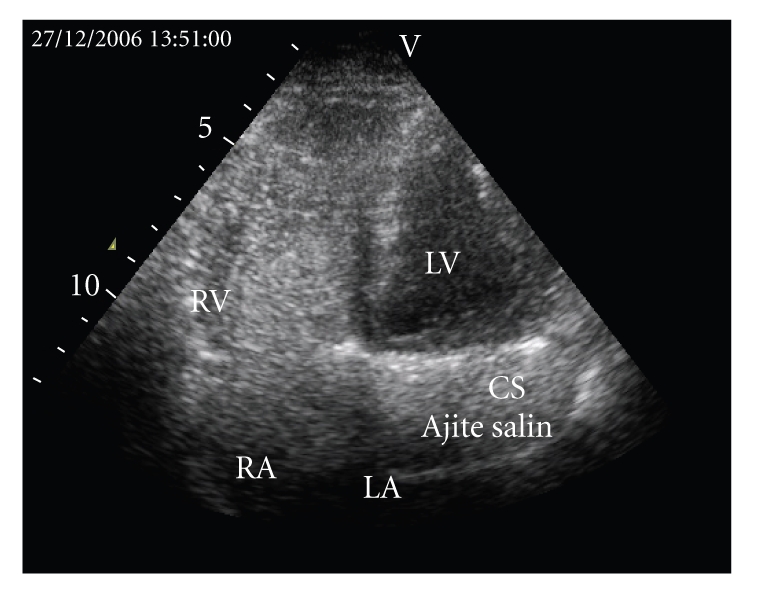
Passage of the contrast into the right atrium via coronary sinus on apical 4 chamber view of transthoracic echocardiography. LA: Left atrium, LV: Left ventricle, RV: Right ventricle, RA: Right atrium; CS: Coronary sinus.
